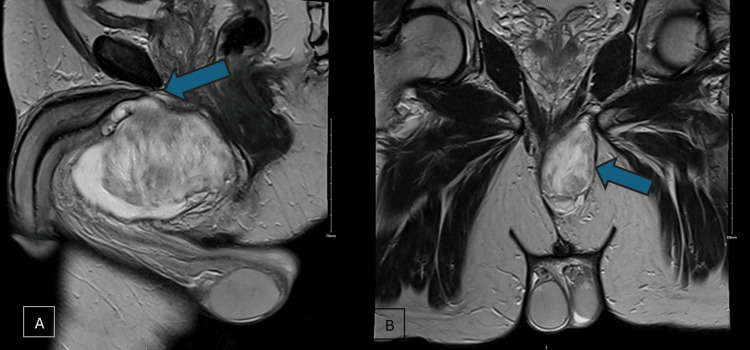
MRI of the scrotum post gadolinium. (A) Sagittal view of the large perineal mass with heterogeneous peripheral enhancement, which is inseparable from the root of the penis (arrowhead). (B) Coronal view of the mass, away from the testis (arrowhead).
